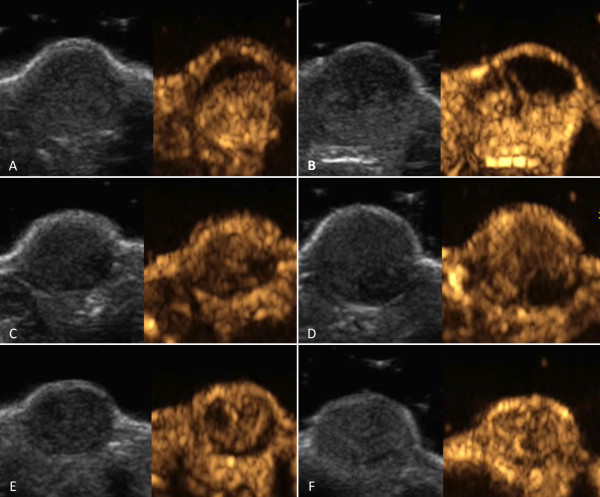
Tumor perfusion at peak enhancement. Representative images of contrast enhanced ultrasonography at peak enhancement in group 1 (panels A and B), group 2 (panels C and D) and group 3 (panels E and F) at day + 5 (panels A, C and E) and at day +13 (panels B, D and F). Corresponding B-mode images are shown. At day +13, necrotic areas are present in group 1 (placebo group) and group 2 (sorafenib-placebo-sorafenib treatment) tumors whereas all tumor areas in group 3 tumors (sorafenib treatment stopped at day +5) are perfused, which might be speculated to derive from overstimulation of neoangiogenesis.
